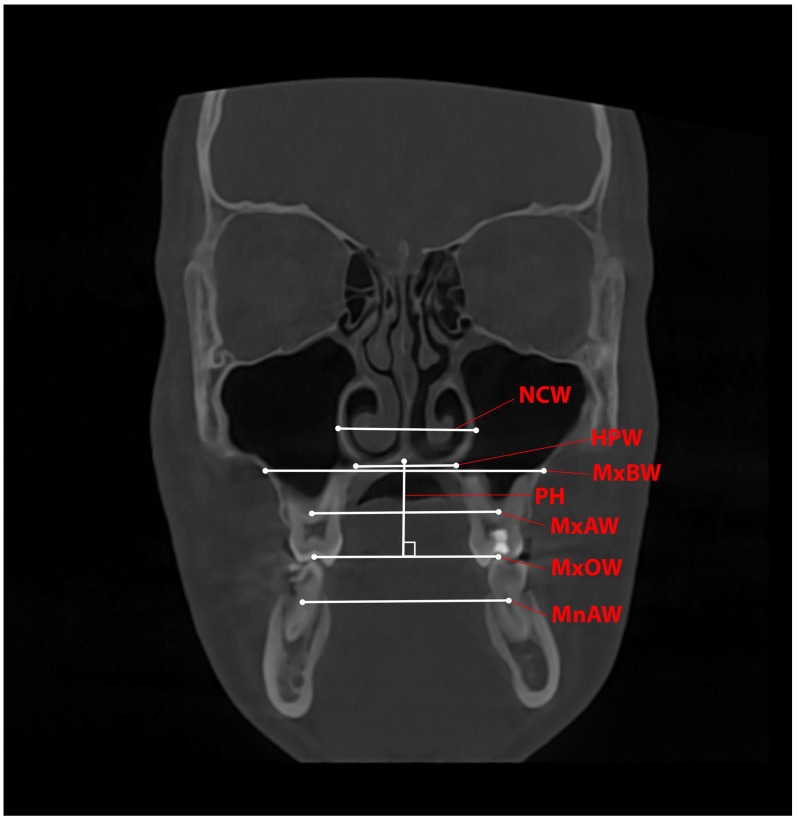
Transverse and vertical measurement of skeletal variables on a CBCT coronal slice. The corresponding parameter is marked in red letters, and its location is marked with a thin red line. The white lines with dots indicate where the parameter is measured.
